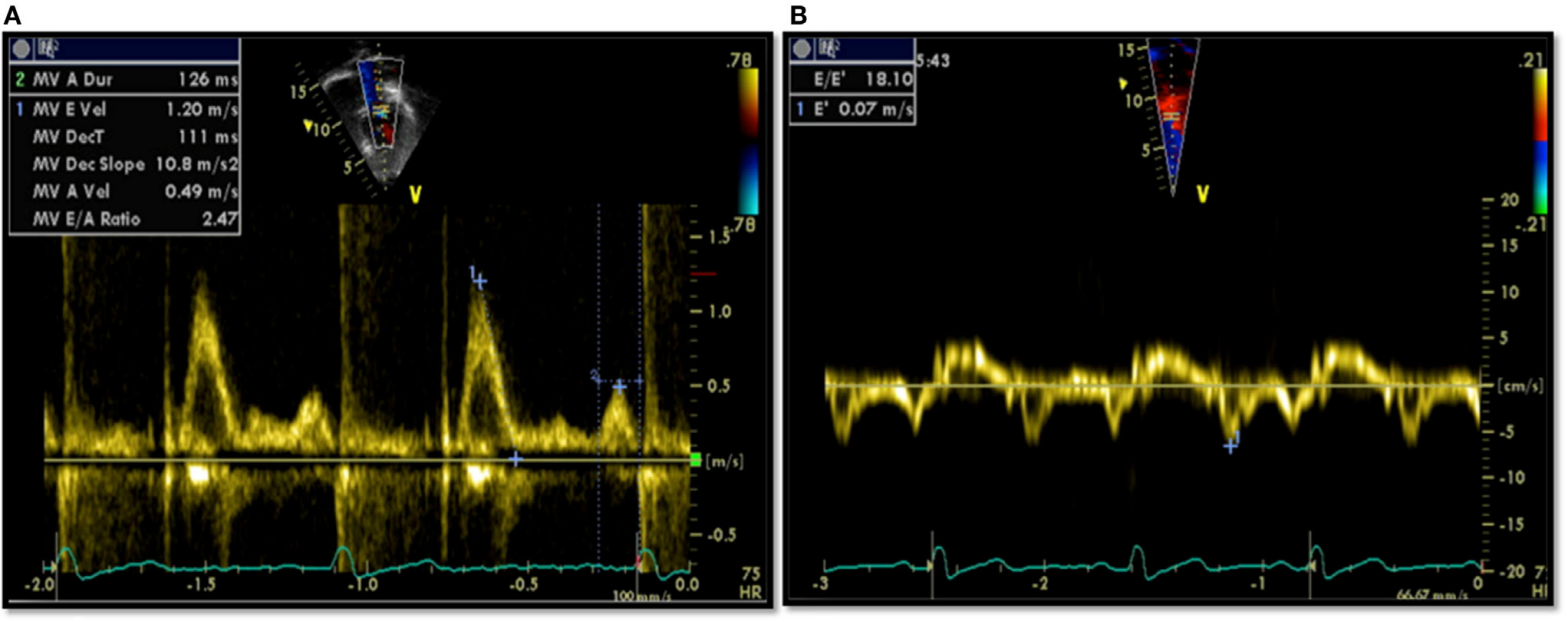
Echocardiographic data from a patient showing diastolic dysfunction: (A) trans-mitral Doppler *E*/*A* = 2.4, deceleration time = 111 ms; (B) tissue Doppler imaging *E*′ = 0.07, a high *E*/*E*′ ratio of 18.1 signifying diastolic dysfunction.
